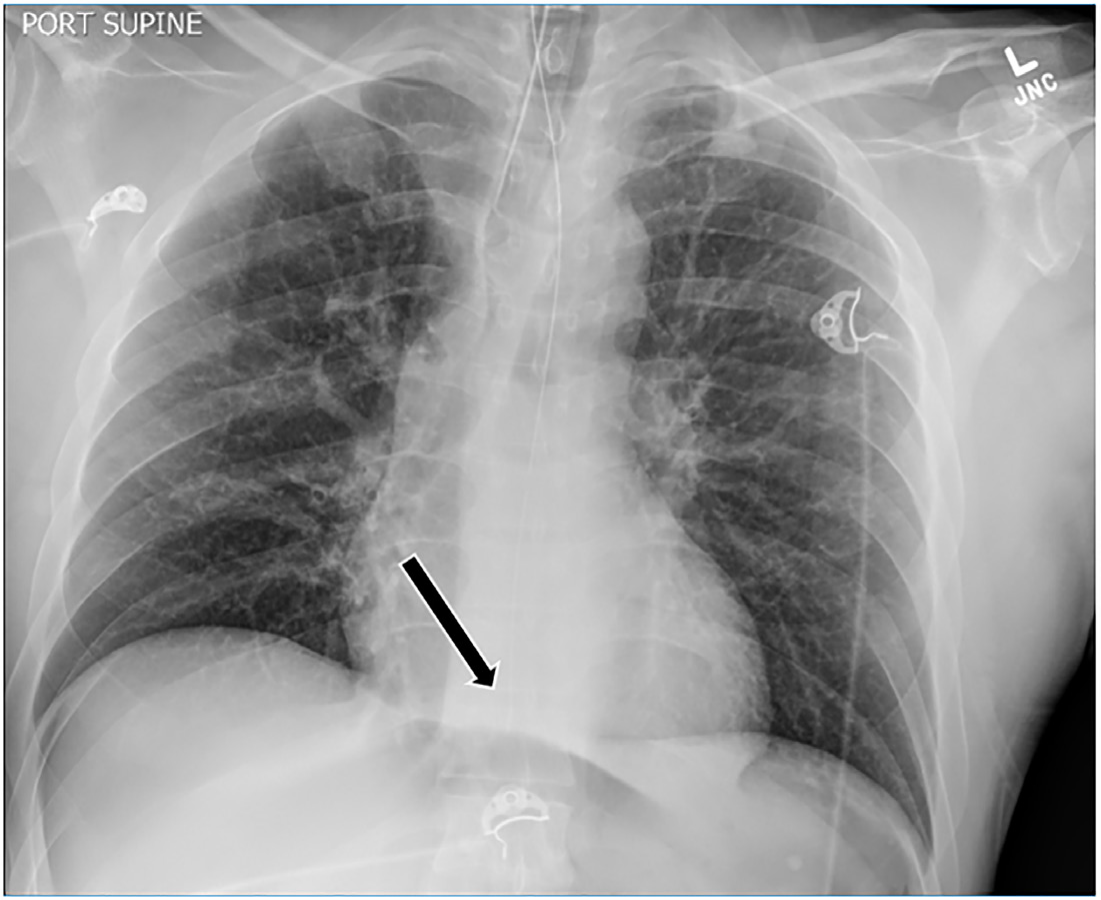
Post-intubation radiograph showing endotracheal tube placement and orogastric tube just superior to the level of the diaphragm, as shown by arrow.
